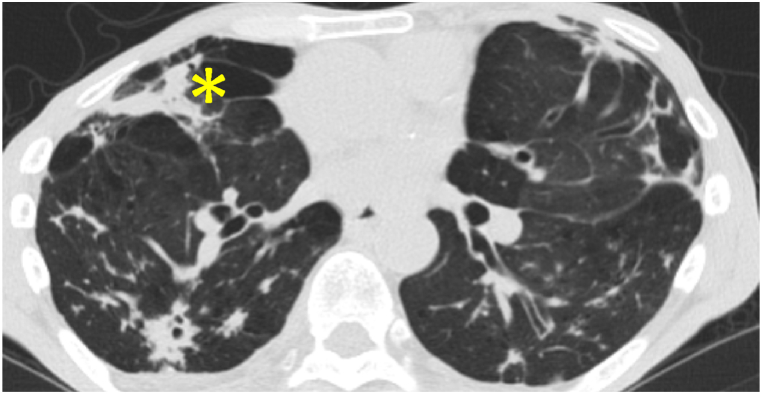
Computed tomography of the chest showing multiple consolidations with cavities, bronchiectasis, and emphysema. *Asterisk* shows a thin-wall cavity of the right middle lobe.
